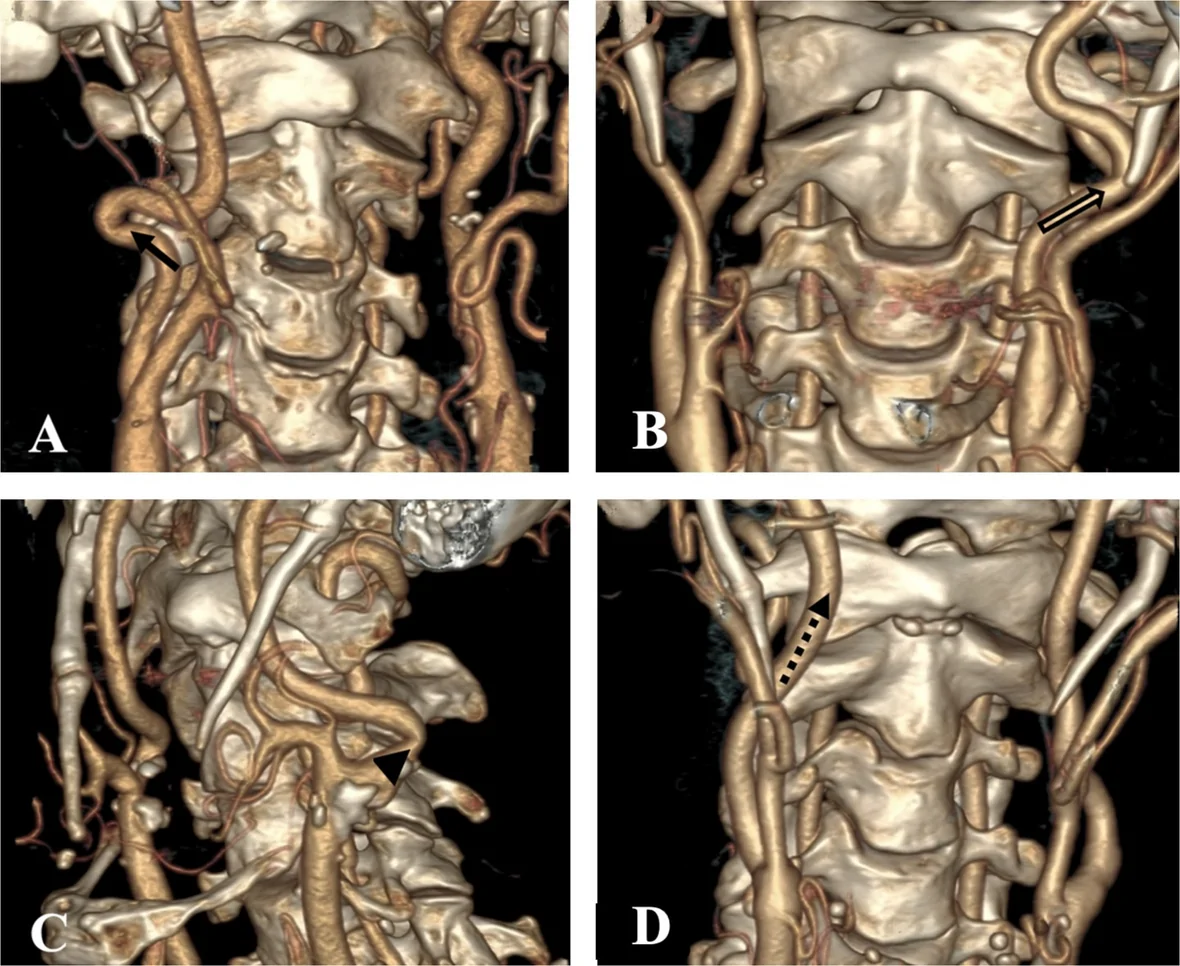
CT angiography of the cervical portion of the internal carotid artery. A and B Kinking pattern (black arrow), C and D: Coiling pattern (black arrow), A and C: Sagittal views, B and D: Frontal views
